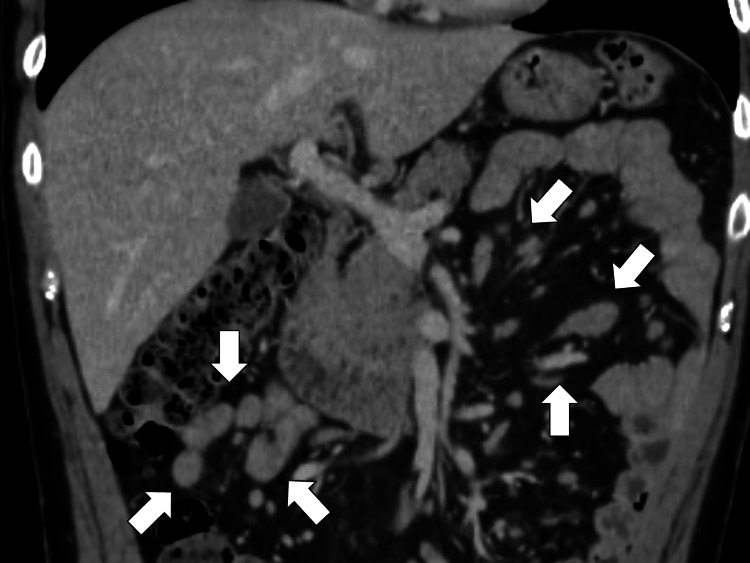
Abdominal computed tomography showing multiple enlarged lymph nodes in the mesentery (white arrows)
